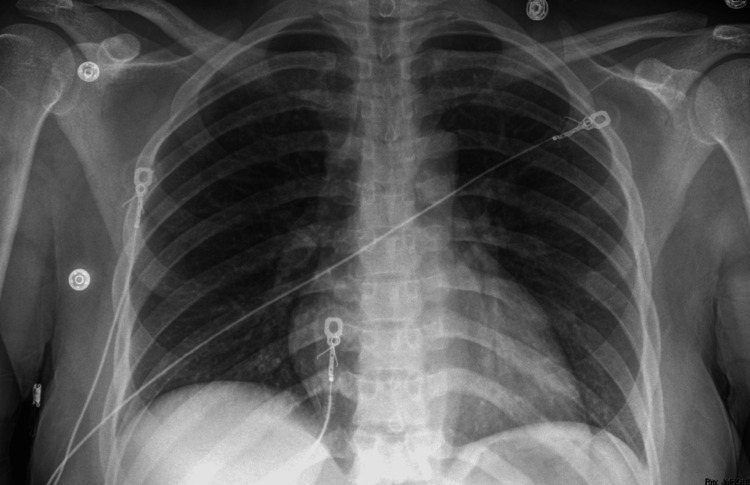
Normal chest radiograph following subglottic stenosis intervention
